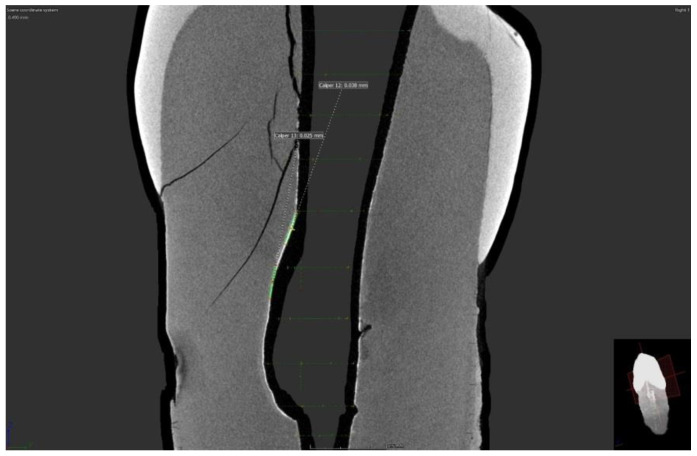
Micro CT scan of sample with removed smear layer.
